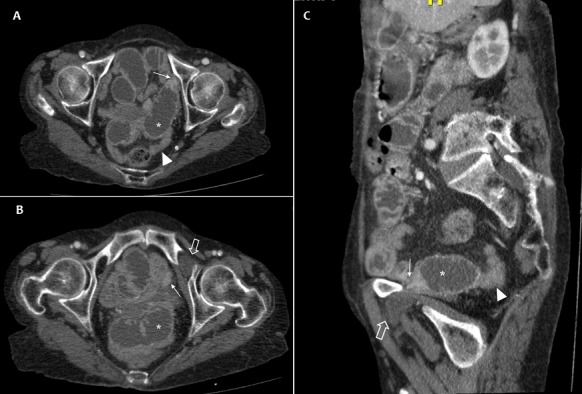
(A) axial pelvic CT scan; (B,C) multiplanar reconstructed images through the left obturator canal, showed a dilated loop of small bowel (asterisk) upstream of a strangulated point (arrow) caused by a left obturator hernia (large arrow). Noted the collapsed loops down and colon (arrowhead)
